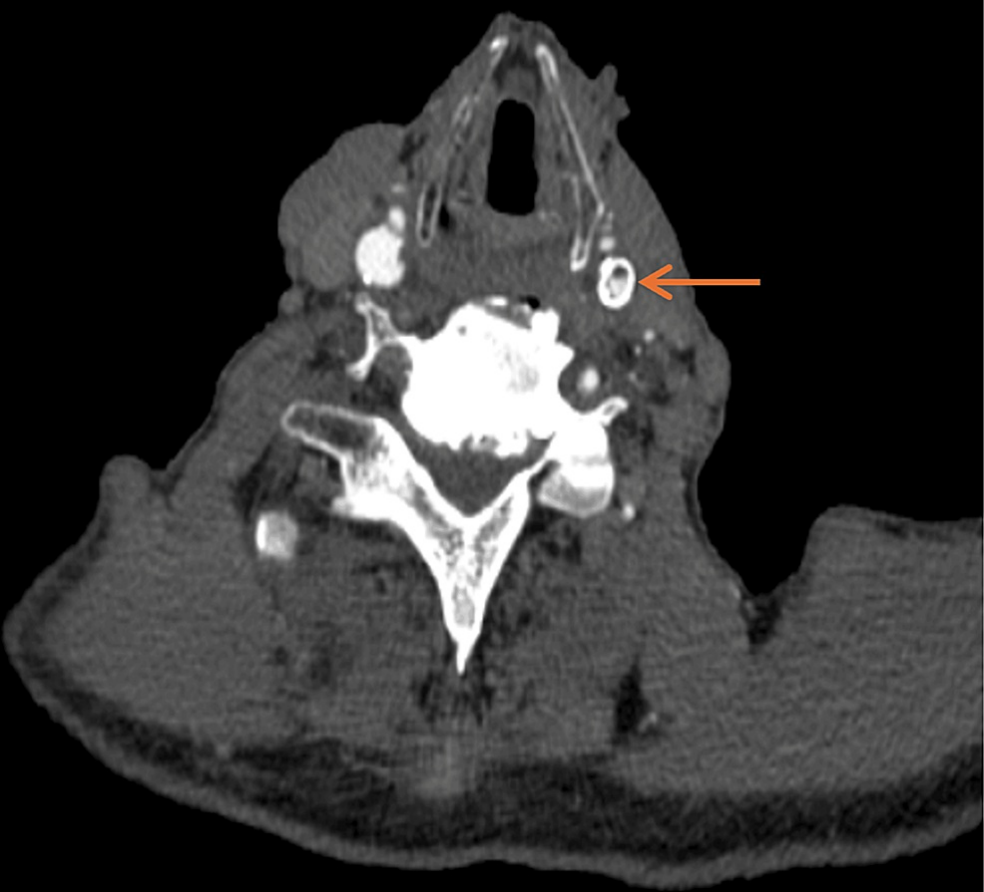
Axial view of a CT angiogram depicting in-stent restenosis of left internal carotid artery stent (arrow).
